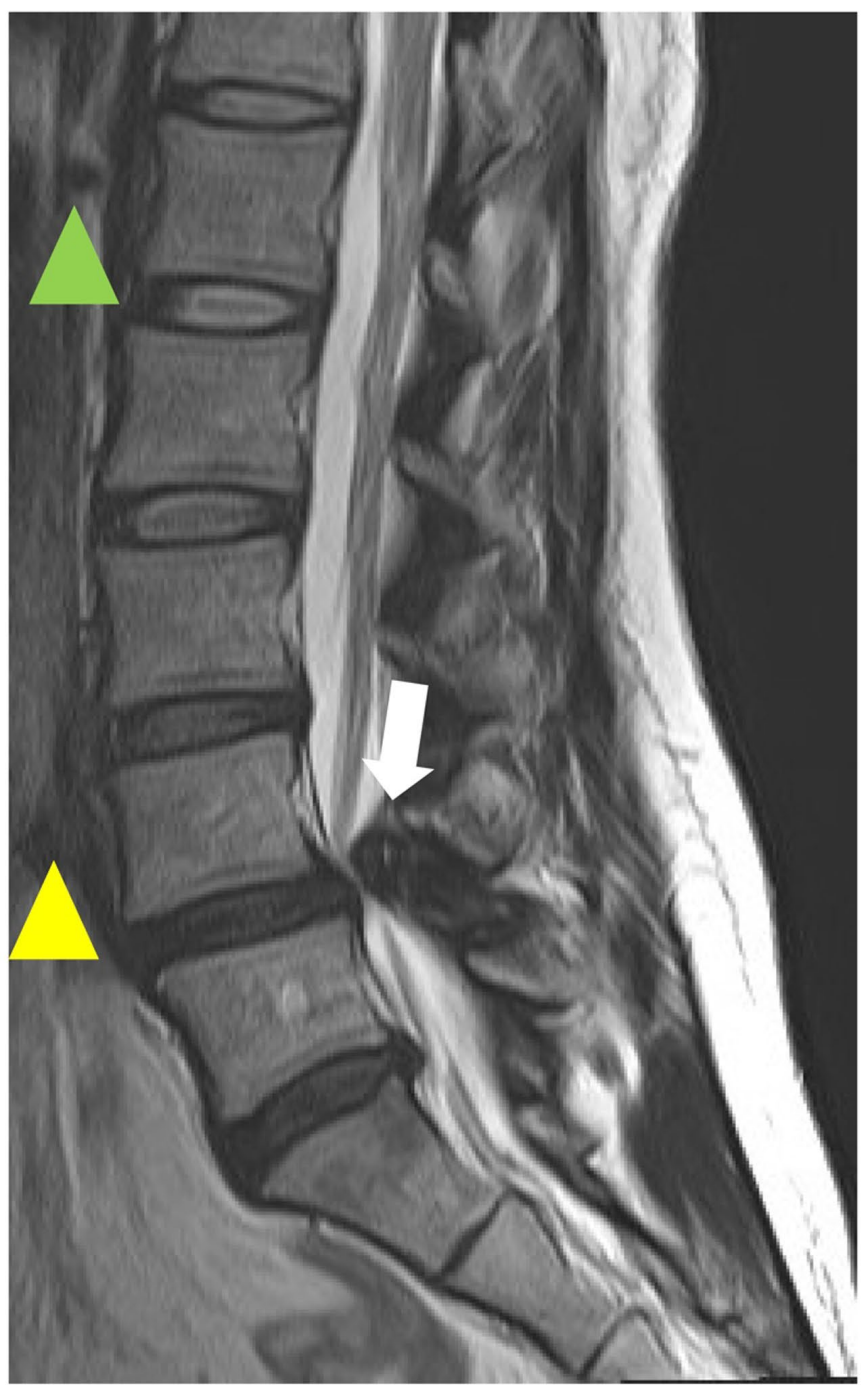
T2-weighted magnetic resonance imaging (MRI) sagittal image of the lumbar spine. The green arrowhead indicates normal intervertebral disk, the yellow arrowhead indicates intervertebral degenerated disk, and the white arrowhead indicates hypertrophic yellow ligament. This is a case from our institution.
